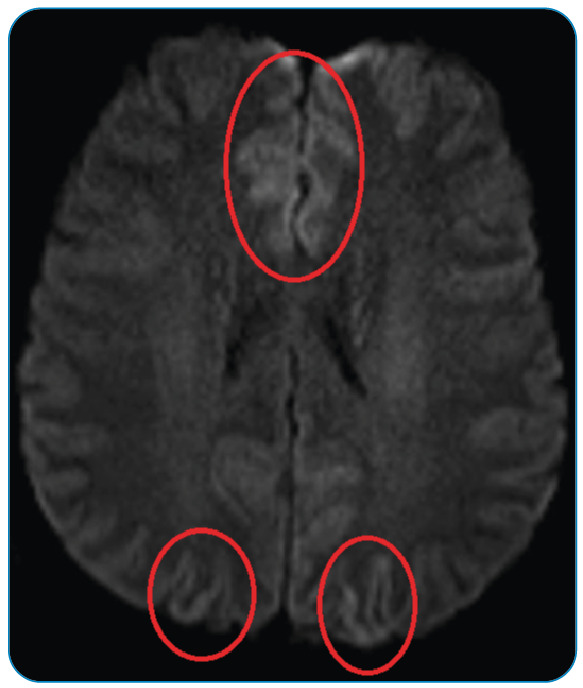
Slightly hyperintense appearances in the cortical gyri of the occipital and frontal lobes on diffusion-weighted MRI
